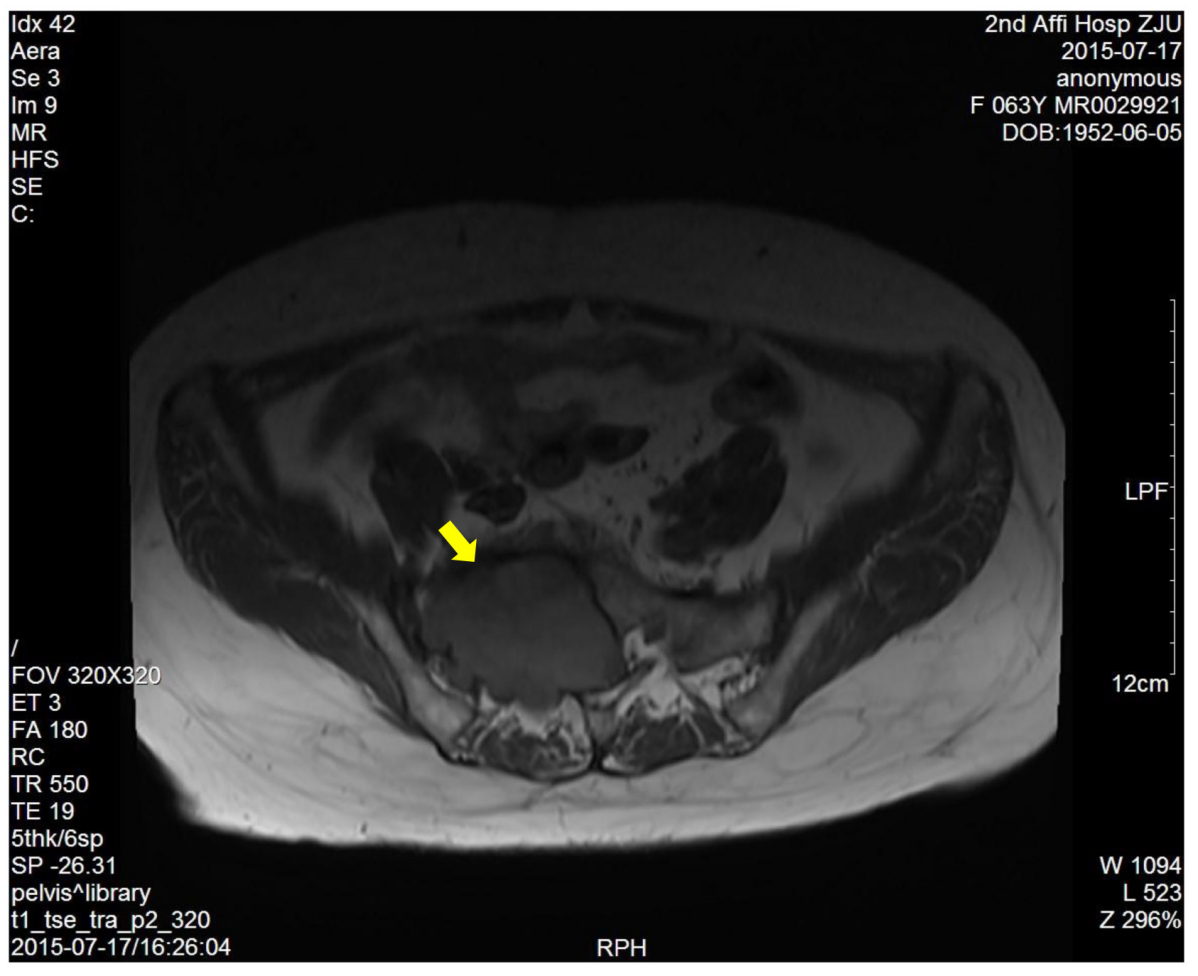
T1-weighted image showed a right sacral mass with low signal intensity
